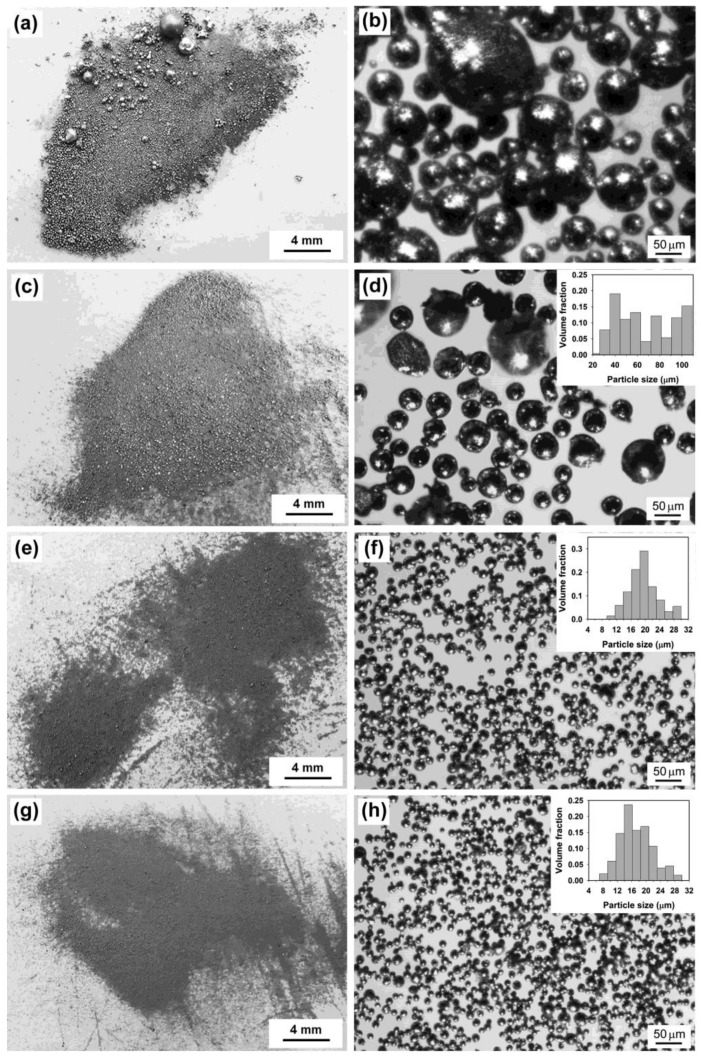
Optical images of the products obtained under different ultrasonic powers: (a,b) 300 W, (c,d) 600 W, (e,f) 840 W, and (g,h) 1080 W. Note that (b) is the image of small Sn particles present in (a). Other experimental conditions: volume ratio of salts to metal = 25:1 and sonication time = 5 min. Inset is the corresponding volume-based particle size distribution.
